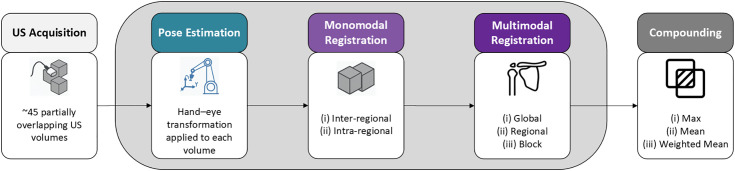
3D US Hybrid reconstruction framework. Partially overlapping US volumes are first positioned using pose-based alignment obtained from robot tracking and hand–eye calibration. Alignment is subsequently semi-automatically refined using monomodal US–US registration followed by multimodal US–MRI registration performed at global, regional, and block levels. The resulting refined alignment is then used for regional and global compounding to generate the final US reconstructions.
